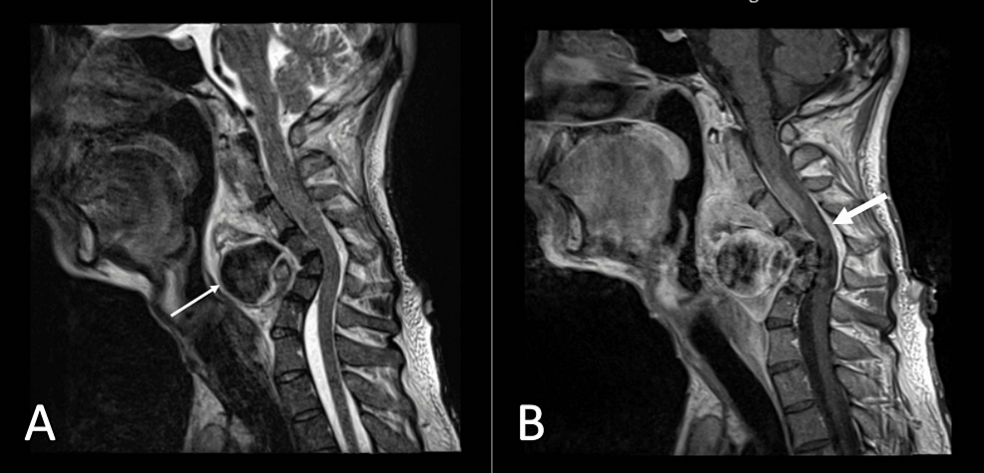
MRI neck. Neck MRI showed T2WI (A) heterogeneous extramedullary lesion (thin arrow) (retropharyngeal region), which was heterogeneously enhanced on post-contrast sagittal T1 (B) predominantly over C2 to C4 with moderate canal stenosis (thick arrow). MRI: magnetic resonance imaging; T2WI: T2 weighted image.
